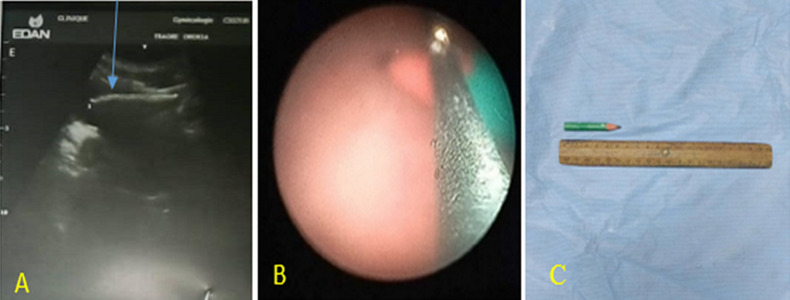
diagnosis and management of intravesical pencil; A) arrow showing foreign body on pelvic ultrasound; B) endoscopic view of the pencil with tripod gripper; C) the removed pencil measuring approximately 50 mm
